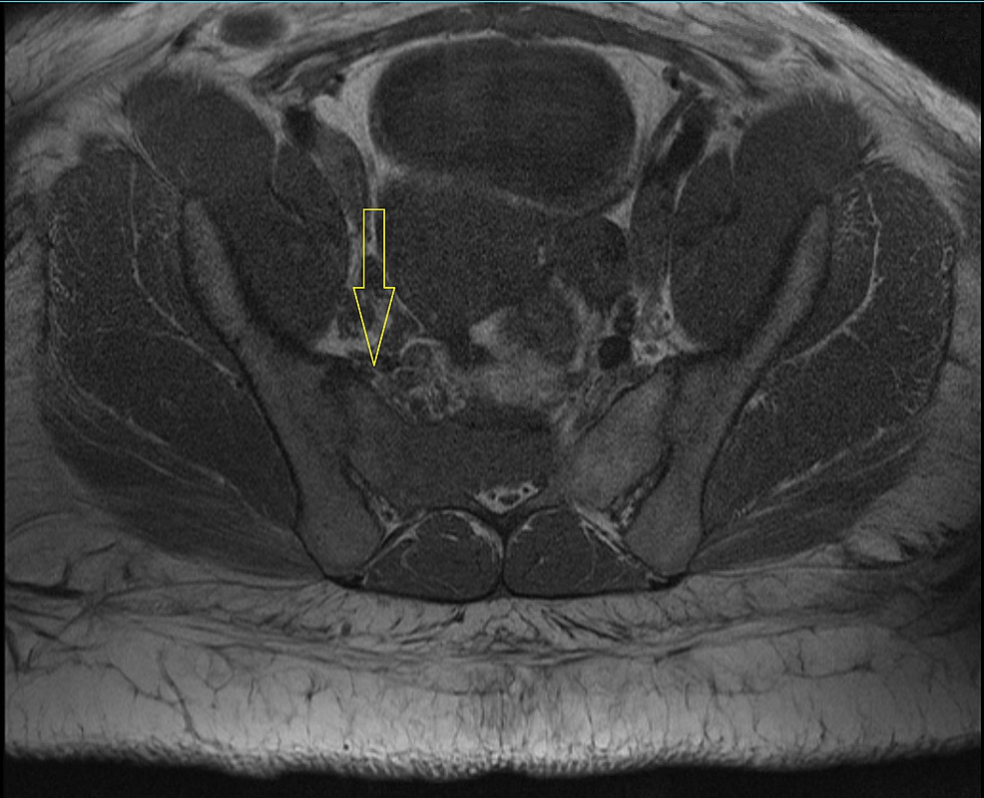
Magnetic resonance imaging of the lumbar spine showing a primarily centrally located sacral mass.
